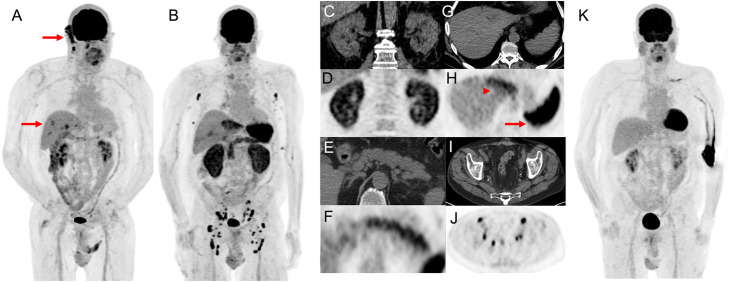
69 year-old man with ulcerated right occipital scalp melanoma, 5 mm deep, mitotic rate of 4 with locoregional nodal metastasis in the right sub-occipital and cervical stations as well as biopsy proven distant metastatic disease in the liver demonstrated on ^18^F-FDG PET MIP (arrows, panel A). Following three cycles of combination ipilimumab and nivolumab presented to emergency department with acute kidney injury (serum creatinine rose from 0.8 mg/dL to 2.8 mg/dL) with progressive fatigue, generalized weakness and poor oral intake. ^18^F-FDG PET MIP (panel B) at 3 months showed resolution of all disease in the right neck and liver and development of FDG-avidity of multiple organs including diffuse parenchymal ^18^F-FDG uptake by both kidneys (panel C and D), diffuse FDG uptake in the pancreas (panel E and F), diffuse ^18^F-FDG uptake in the left lobe of the live (arrow heads, panel G and H), marked diffuse ^18^F-FDG uptake in the spleen (arrows, panel G and H) as well as bilateral axillary, iliac and inguinal lymphadenopathy (panel I an J) suggestive of, interstitial nephritis, pancreatitis, likely cholangitis and granulomatous disease, respectively. The patient discontinued ICIs and was treated with high dose corticosteroid and all findings resolved at 5 months follow-up with gradual improvement in serum creatinine (Panel K)
